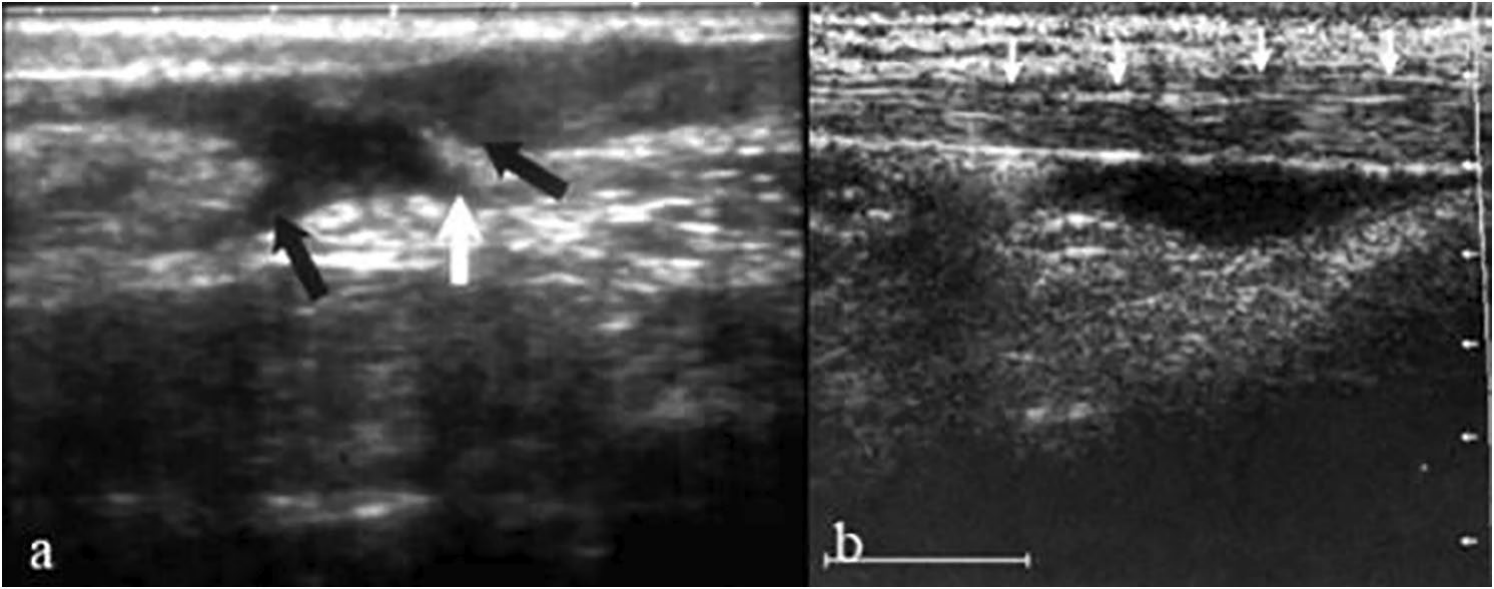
a Intra-operative ultrasound before repair (black arrows represent torn edges and white arrow represents sural nerve. b Intra-operative ultrasound after repair with good contact between torn edges
